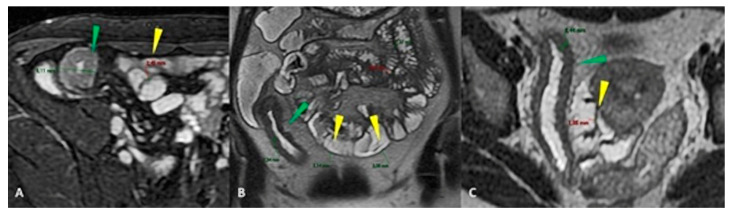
Axial (A) FIESTA sequence: Wall thickening of 9mm (green arrowhead). Coronal T2-weighted (B,C) wall thickness (green arrowhead in B,C). Normal wall thickness <3 mm (yellow arrowhead in A–C).
